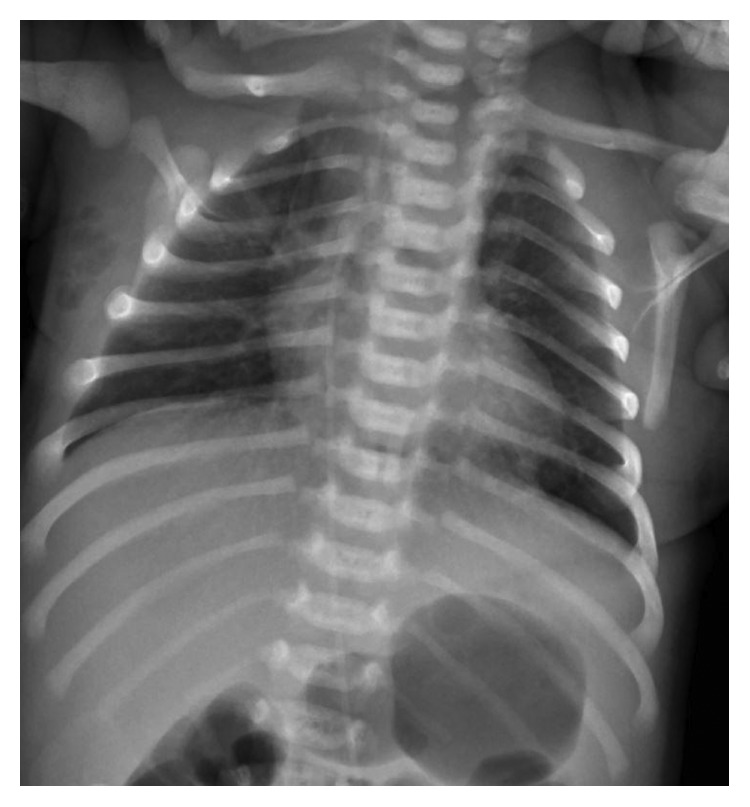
Chest radiograph on day 5 showing resolution of pneumothorax and pneumopericardium, with residual subcutaneous emphysema over right axilla.
